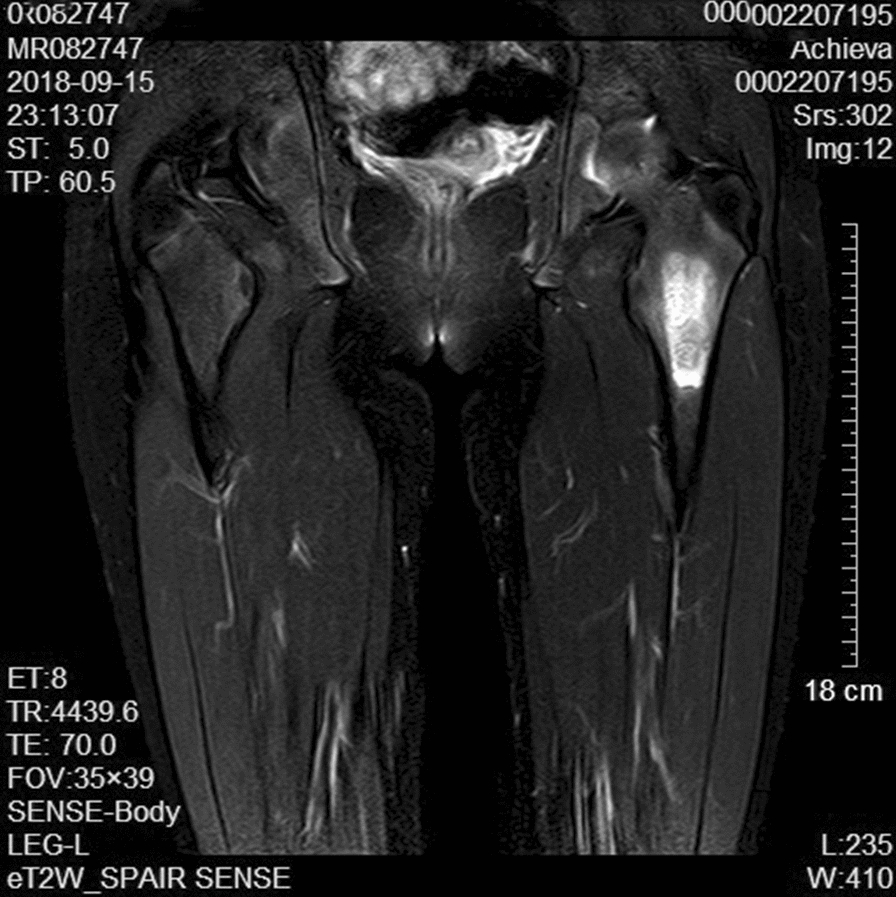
Preoperative magnetic resonance imaging (MRI) showed an oval mass that was hypointense in the T1-weighted image and hyperintense in the fat-suppressed T2-weighted image in the bone marrow cavity of the proximal femur
